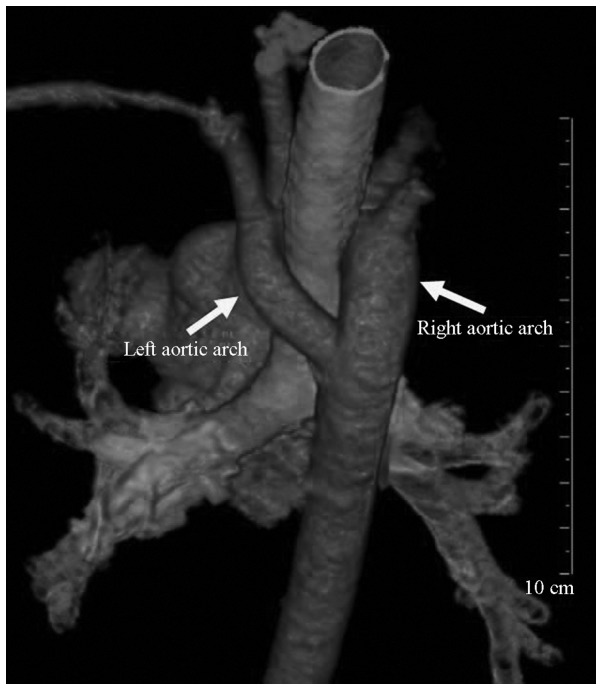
A ten-month-old male infant with DAA. The CT volume-rendered image shows that the aortic arch split into two arches surrounding the trachea (thick right arch and small left arch). DAA, double aortic arch; CT, computed tomography.
